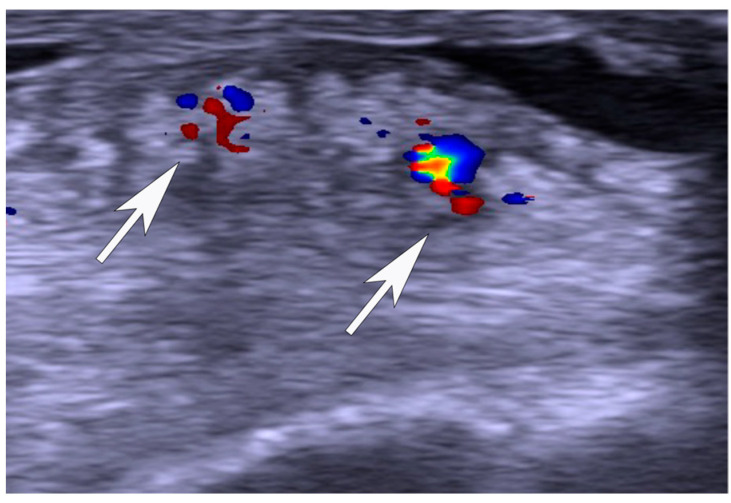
Ultrasound and Doppler image that shows enlarged and thickened subcutaneous bursa with localised high blood flow in deep side of the bursa (arrows).
